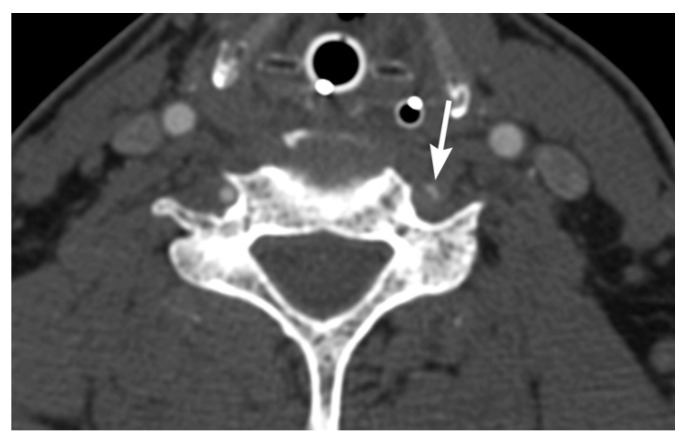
59-year-old male with left vertebral artery injury. Axial multidetector CT angiographic image shows severe eccentric crescentic left vertebral artery narrowing from dissection.
